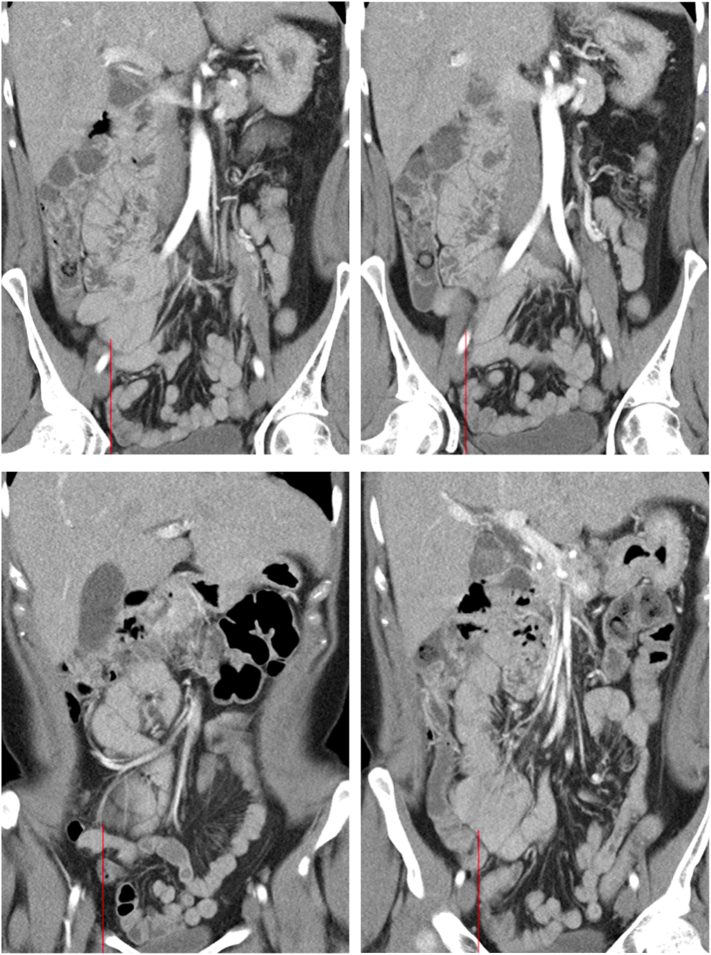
CT images from prior ED visit 2 months before hospital admission for abdominal pain with no mention of malrotation.
